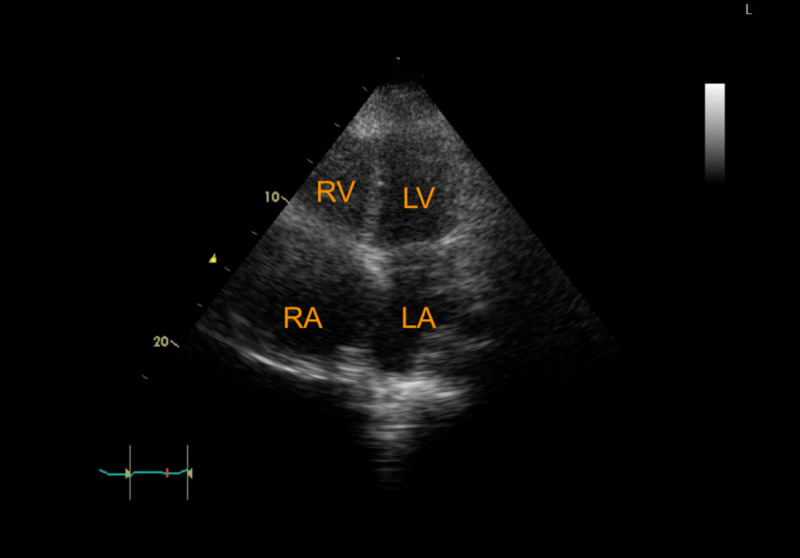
Postoperative echocardiogram with no pericardial effusion Apical four-chamber view showing RA, RV, LA, and LV LA: left atrium; LV: left ventricle; RA: right atrium; RV: right ventricle
